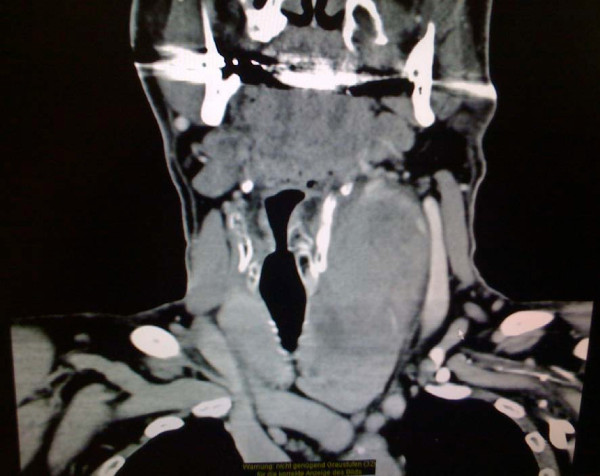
Coronal computed tomography (CT) image showing the possible connection of the mass to the thyroid gland.
